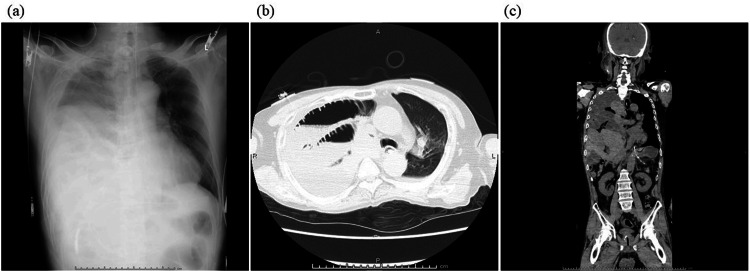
Chest X-ray and CT scan on POD 6. (a) Chest X-ray showed decreased right lung permeability. (b, c) CT scan showed that the dilated intestinal tract was fitted into the right thoracic cavity. CT, computed tomography; POD, postoperative day
